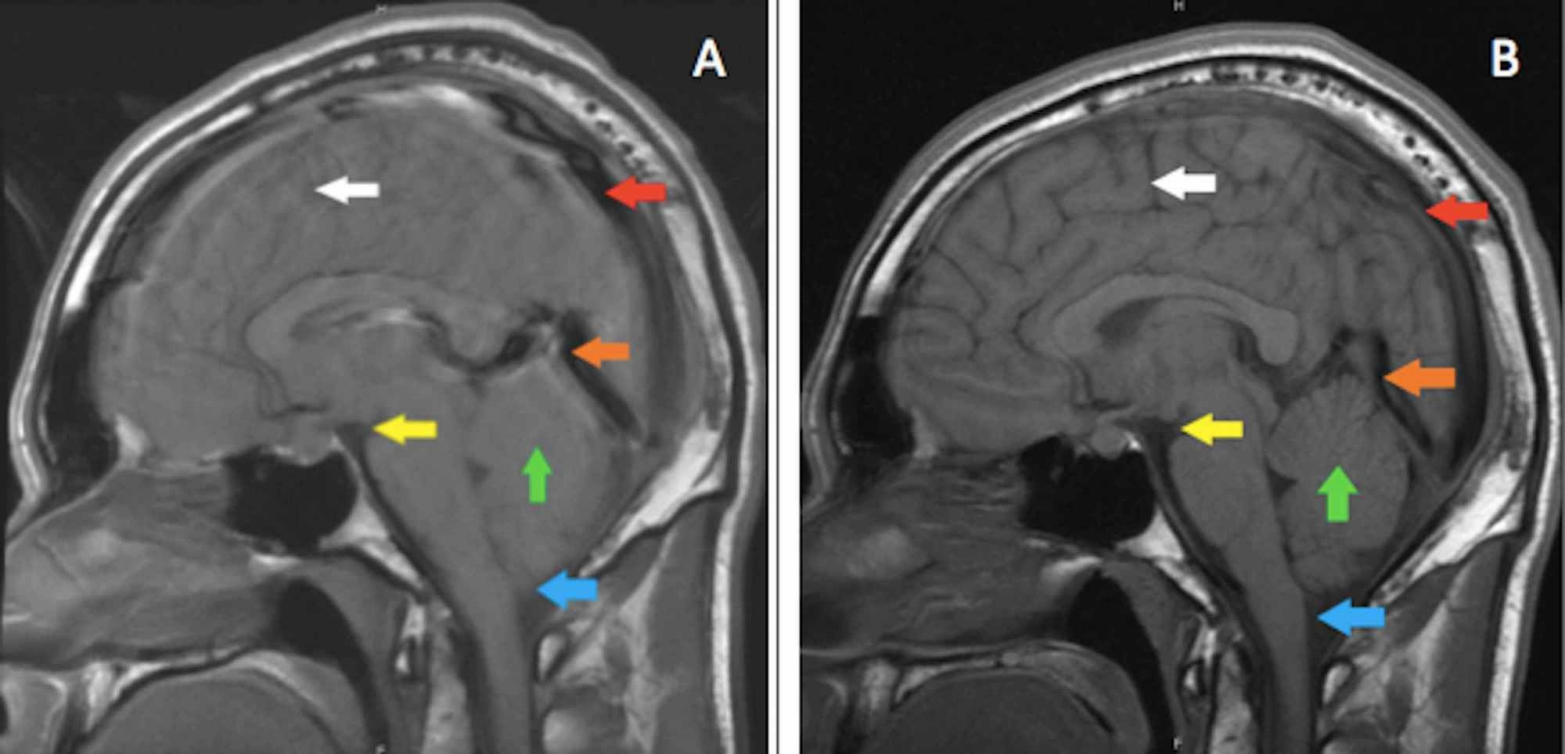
Brain MRI with findings of intracranial hypotension (A) Initial brain MRI with findings of intracranial hypotension prior to blood patching. Significant findings include compression of cortical gyri (white arrow), increased space inside the cavity (red arrow), venous distension (orange arrow), decreased mamillopontine distance (yellow arrow), compression of the cerebellar gyri (green arrow), and a "low riding" brainstem at the foramen magnum (blue arrow). (B) Image shows improvement of the aforementioned findings at week 34 of injury, after blood patch treatments.
